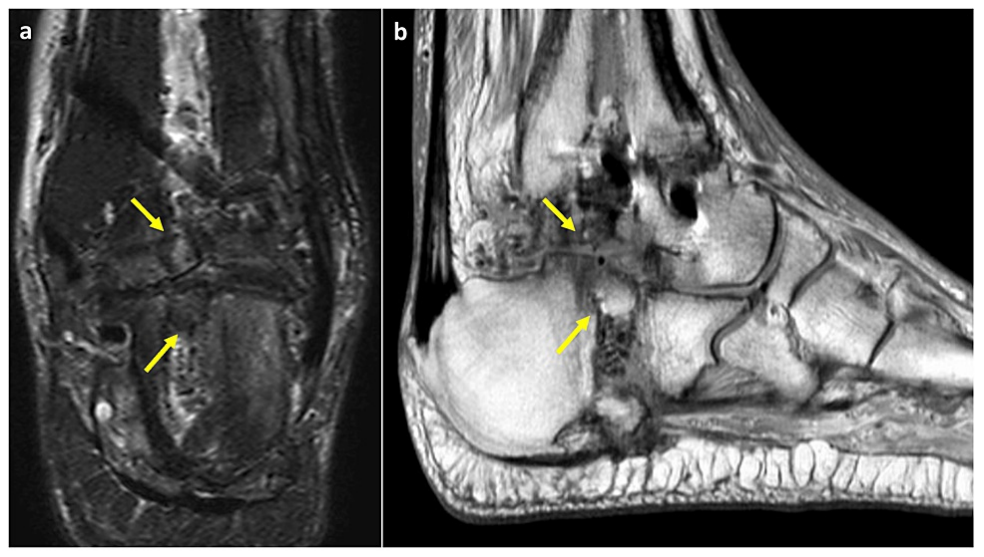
MRI at six months after surgery (a) coronal image of the T2-weighed fat suppression and (b) sagittal image of the proton density MRI, magnetic resonance imaging
